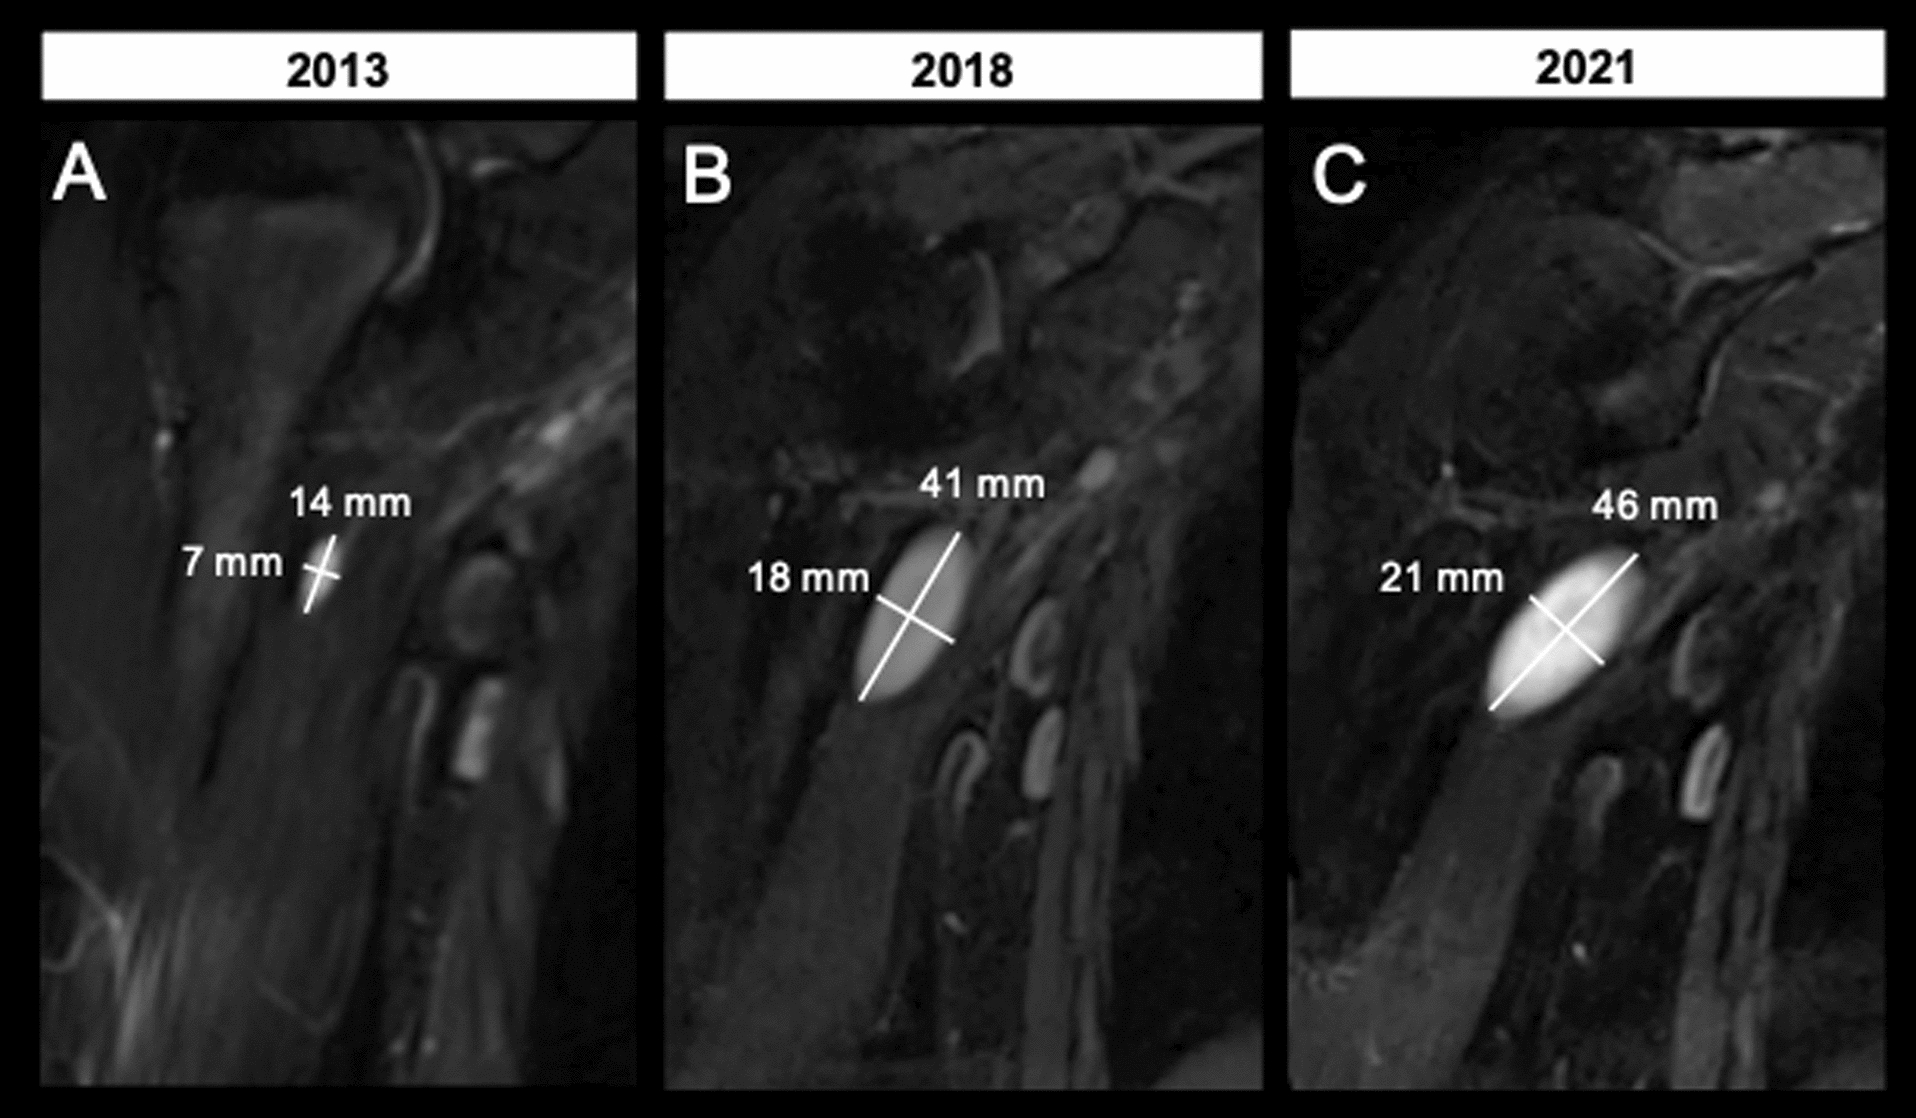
Long-term tumor growth characteristics of the distinct nodular lesion in the right upper arm of patient example 1. A Coronal T2-weighted, fat-saturated MRI of the right upper arm of a 16-year-old male, acquired at 1.5T in 2013 showed a newly developed lesion measuring 14 mm × 7 mm. B Follow-up examination in 2018, acquired at 3T at the age of 21, revealed an increase in size to 41 mm × 18 mm (growth of 192.9% in the long axis). C Follow-up examination in 2021 acquired at 3T at the age of 24 showed an increase in size of the lesion to 46 mm × 21 mm (percentage growth of 12.2% in the long axis). Assuming continuous growth pattern, the median tumor growth rate per year was 12.5%
